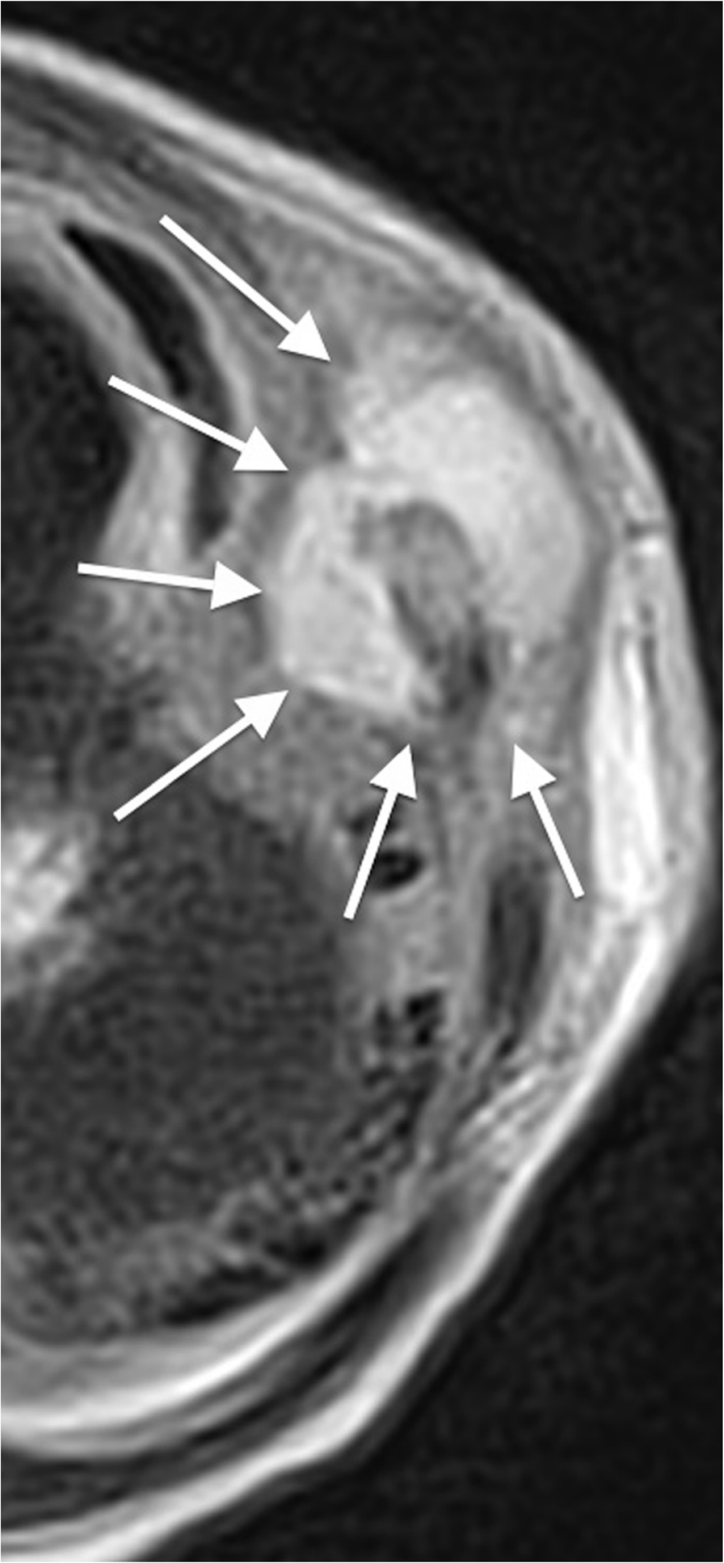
Rib osteomyelitis: magnetic resonance imaging. The axial T2-weighted image shows a fluid collection with high signal intensity, encasing the anterior portion of the seventh left rib (white arrows). The rib is deformed and fragmented, with bone marrow hyperintensity
